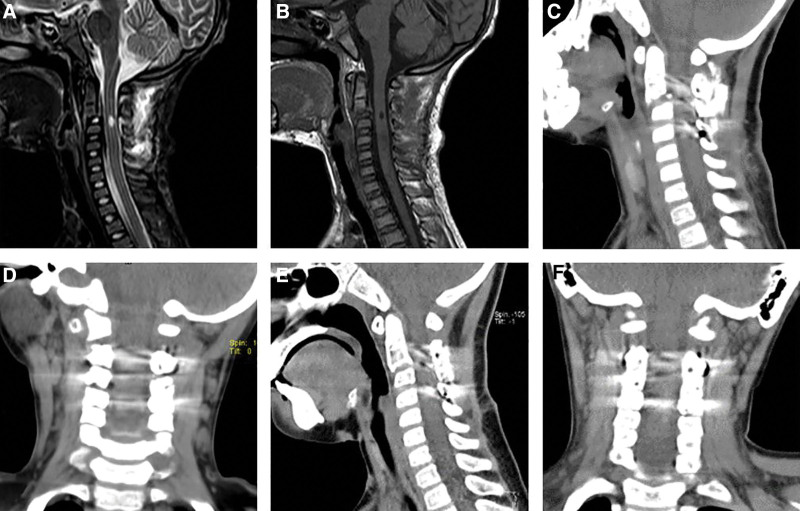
The postoperative cervical MRI (A and B) showed that the spinal cord injury at the C2/3 intervertebral space level was better than before. The postoperative cervical CT (C and D) showed that C2 vertebral body forward slippage was significantly improved, spinal canal stenosis in the corresponding segment was reduced, the cervical spine sequence was well recovered, and screws were accurately implanted into the pedicle.
